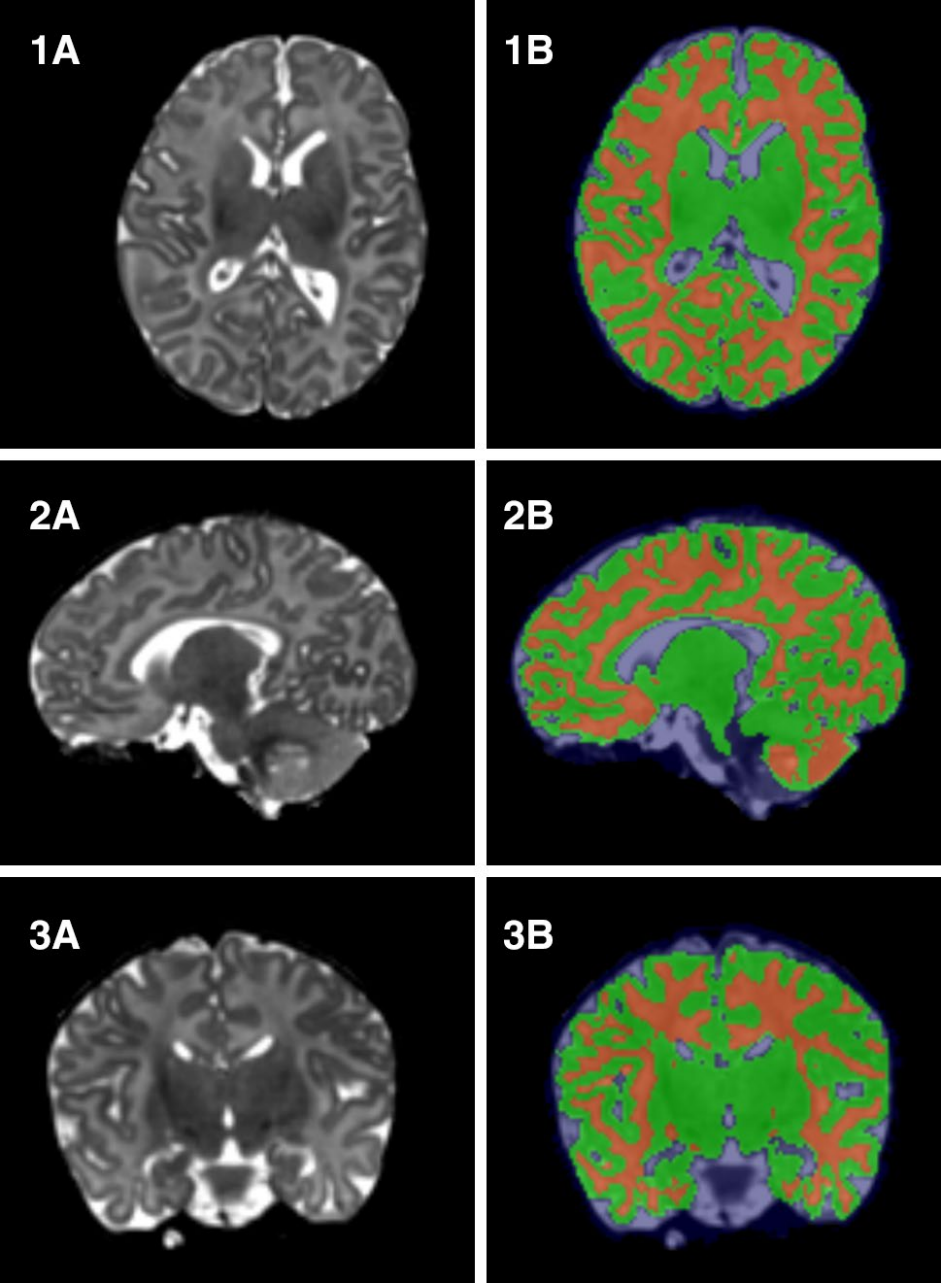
Representatives of (1) sagittal, (2) axial and (3) coronal views generated from a subject’s T2-weighted image. 1A–3A are the original T2-weighted images and 1B–3B are the segmented images, where the gray matter is marked with green and the white matter by red
